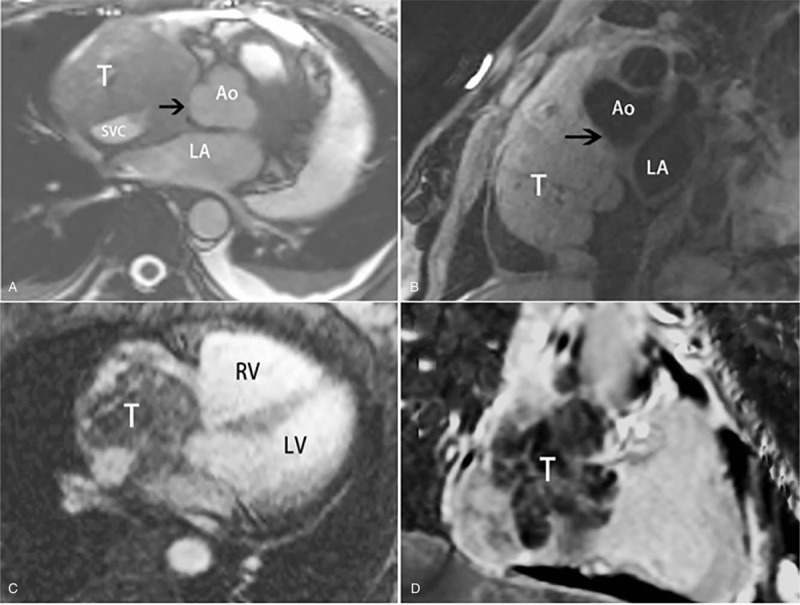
MRI features of the malignant peripheral nerve sheath tumor in right atrium. (A) Bright blood sequence, (B) T2-weighted images show an isointense and slight hyperintense mass attached to atrial right wall with a broad base, respectively. The fat plane between the mass and aorta is not clear and SVC is compressed. (C) First-pass perfusion demonstrates an ill-defined mass with fast perfusion. (D) The mass shows heterogeneous delayed contrast enhancement after gadolinium administration. Note: abbreviations as in Figure 1.
